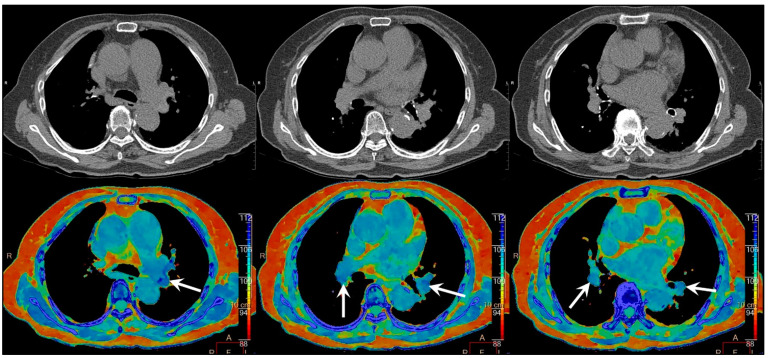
Upper row: unenhanced chest CT images in mediastinal window setting showing unremarkable findings. Lower row: color overlayed electron density images showing multiple high-density ED areas in both pulmonary arteries (arrows). Scale bar is shown on the right. Materials with higher electron densities are shown in dark blue. Lower-density materials are shown in light blue or yellow. ED, electron density.
